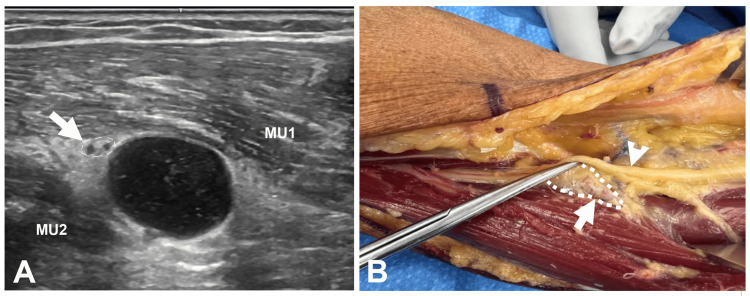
Finger Drop Caused by Compression by a Ganglion Cyst (A) Ultrasound: short axis view at the lateral elbow showing the PIN (arrow) with two fascicles (hypoechoic dots within a circle). MU1: extensor digitorum muscle; MU2: extensor carpi ulnaris muscle. (B) The patient underwent neurolysis of the radial nerve, excision of the ganglion cyst, and elbow arthrotomy. The intraoperative photo shows the cyst (oblique arrow, within a circle) and the overlying PIN (vertical arrowhead). PIN: posterior interosseous nerve
